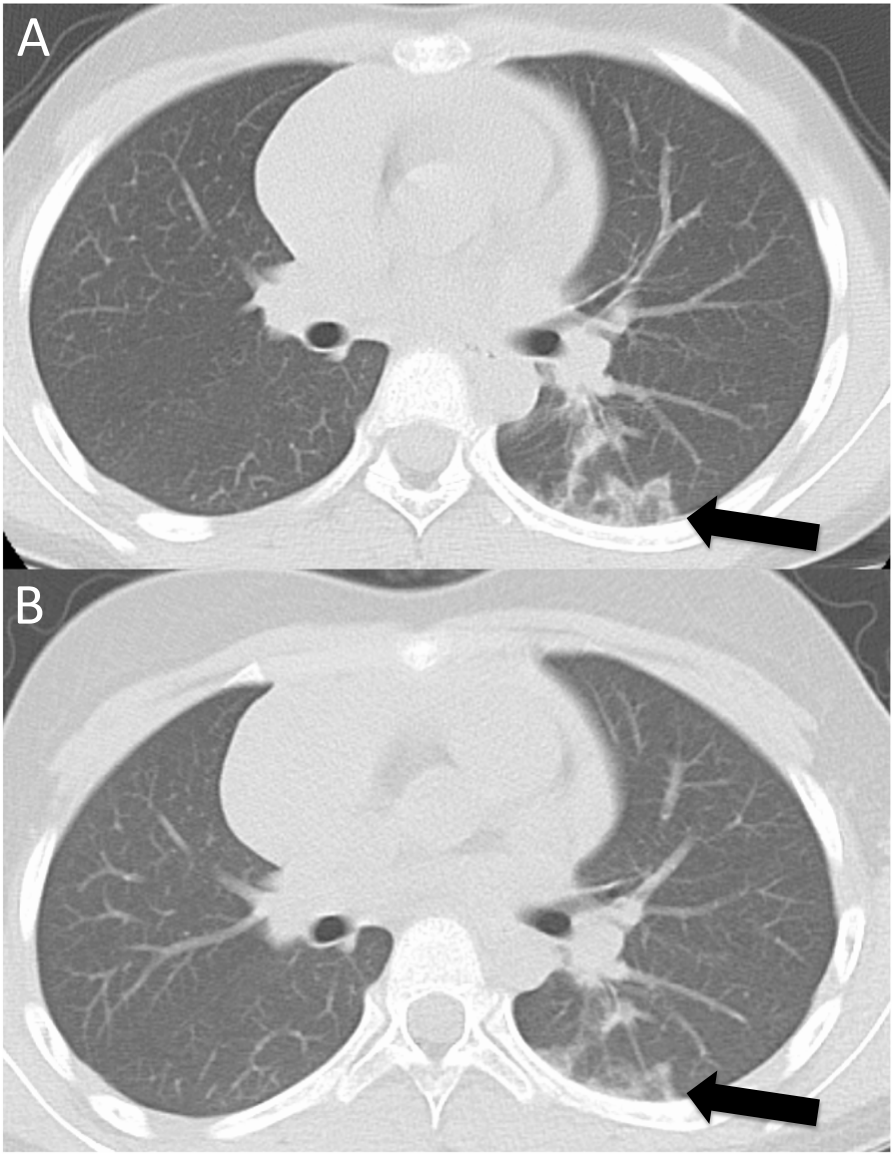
Chest computed tomography (CT) findings. a A ground-glass opacity was observed in the left segment 6 under the visceral pleura before treatment. The arrow shows the ground-glass opacity. b Ground-glass opacity was slightly improved but not progressive 18 months after treatment initiation. The arrow shows the ground-glass opacity
